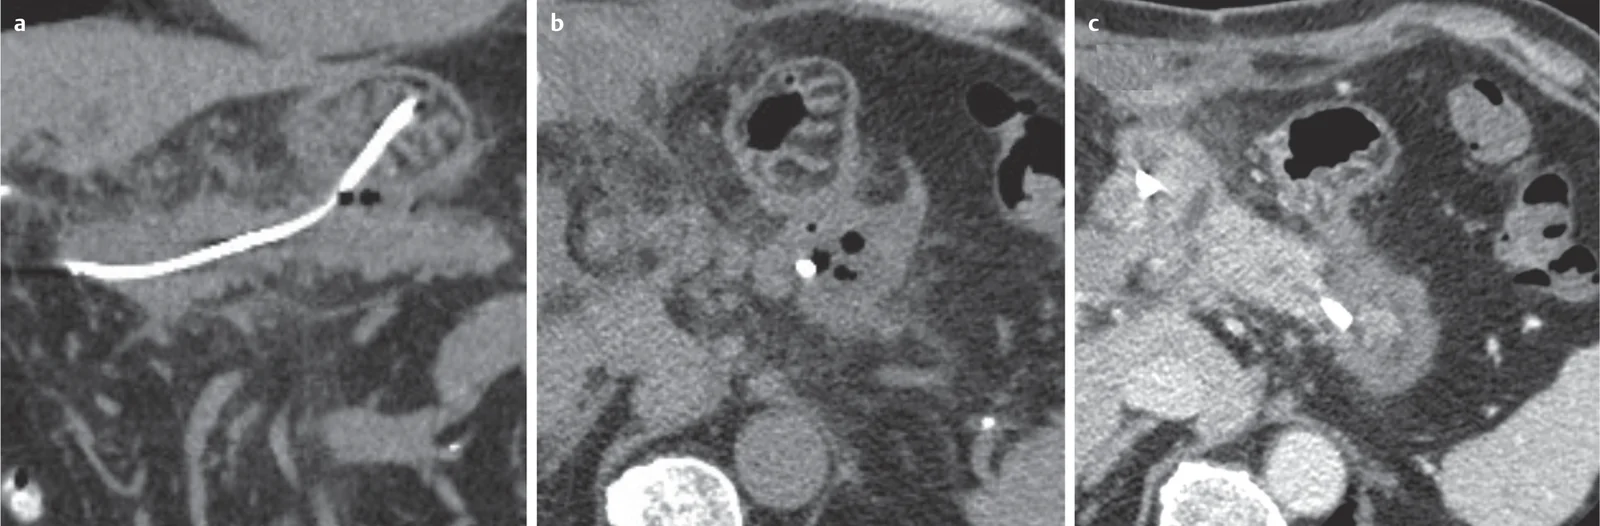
Postprocedural computed tomography findings. (
